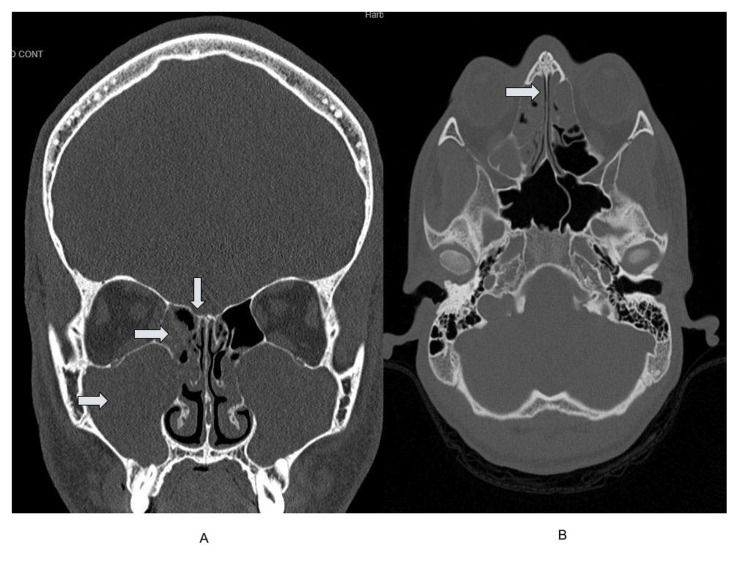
Admission (symptom day 21) computed tomography of the maxillofacial sinuses without contrast. (A) Complete opacification of both frontal and maxillary sinuses. (B) Opacification of ethmoid sinuses.
